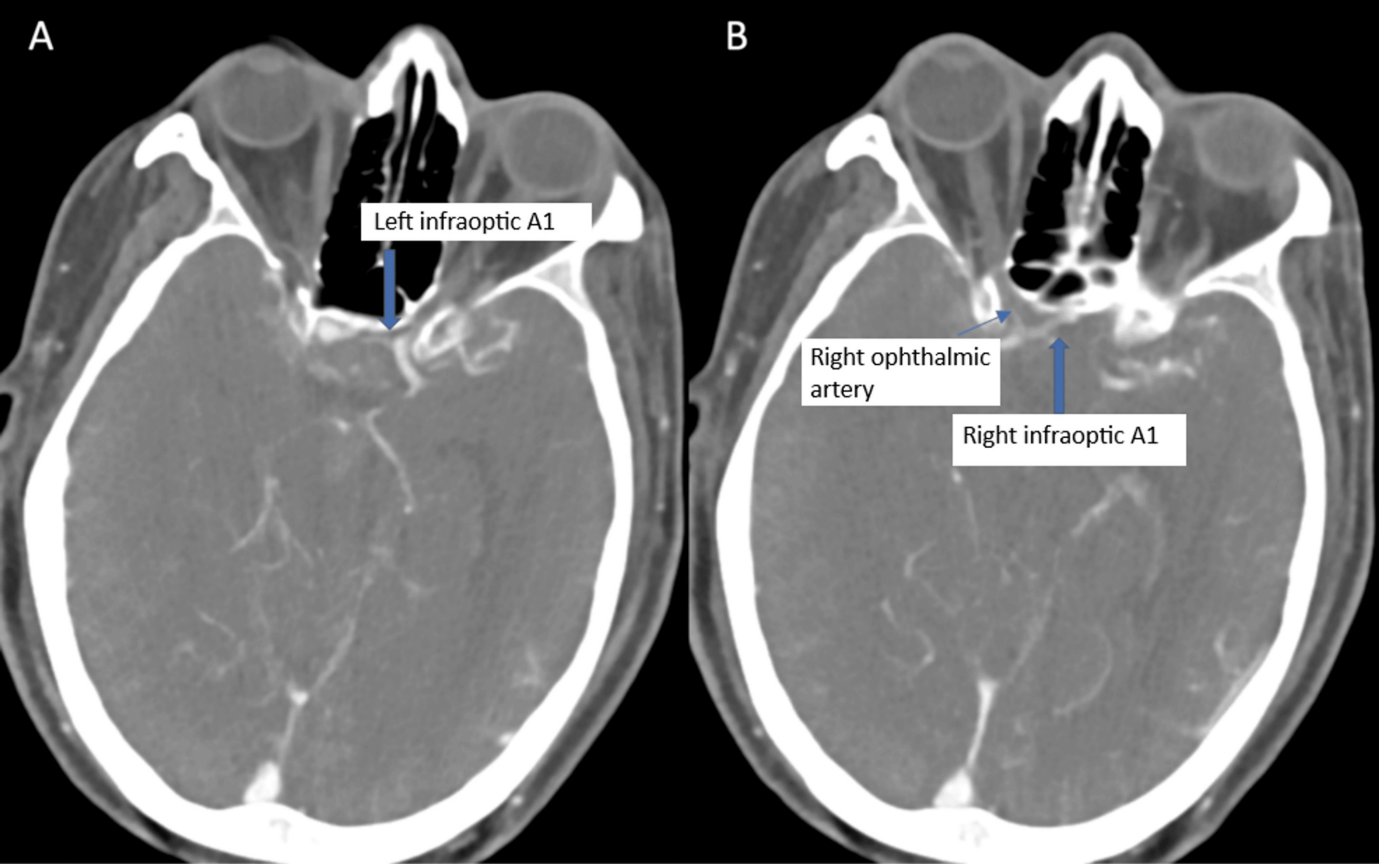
Axial CT cerebral angiography demonstrating bilateral infraoptic courses of the anterior cerebral arteries (ACAs) (A) Left infraoptic A1 segment (blue arrow). (B) Right infraoptic A1 segment (blue arrow) and right ophthalmic artery (blue arrowhead). The A1 segments arise from the internal carotid arteries (ICA) and course beneath the optic nerves before turning medially toward the anterior communicating artery (ACoA) complex.
